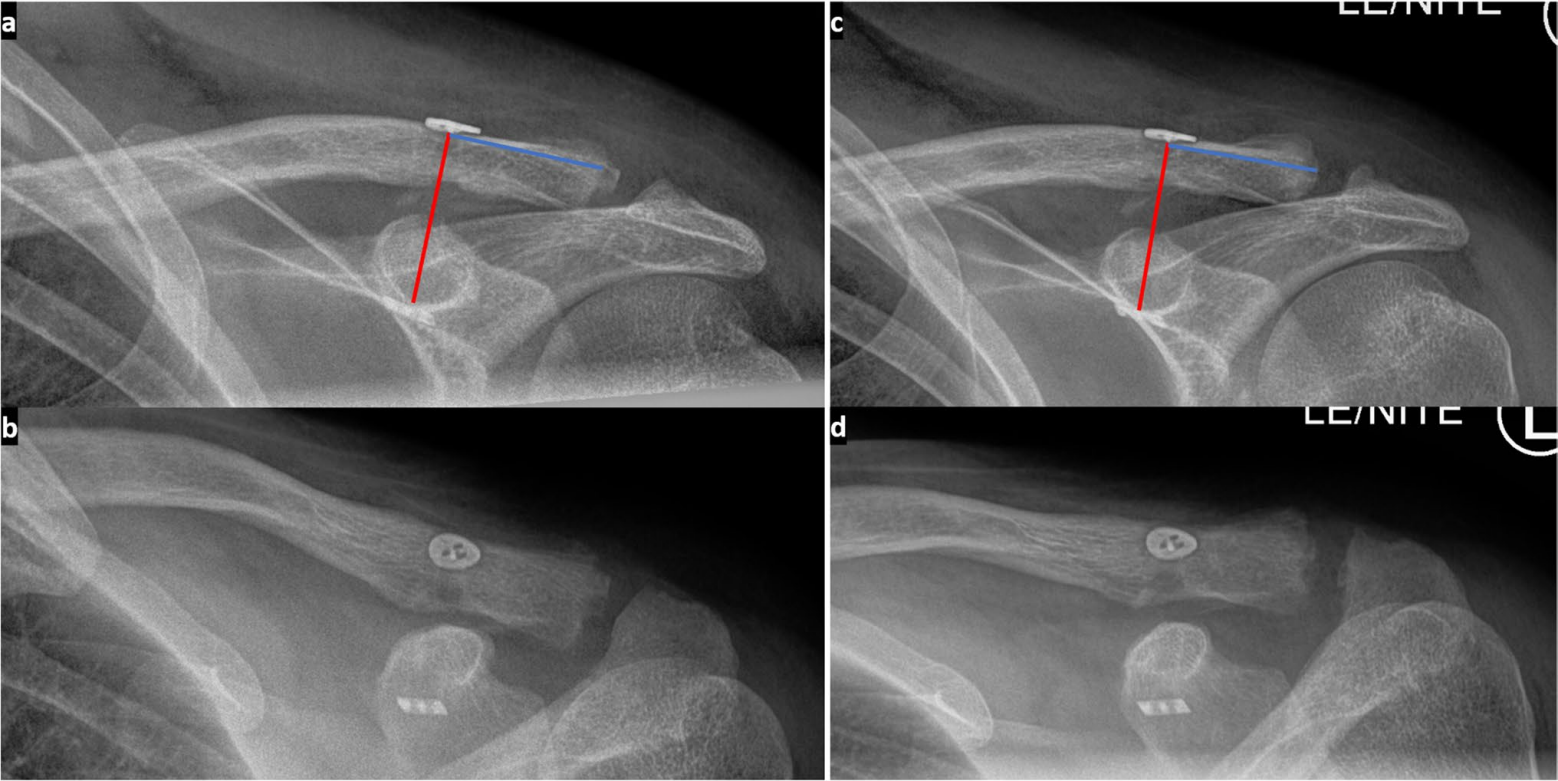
The collection of radiological measurement data is demonstrated here on two X-ray controls in two planes each. The anterior posterior (a.p. / coronal) AC joint images at discharge (a) and after 3 months (c) show the measured CC distance (vertical AC joint congruence) between the titanium plates in mm (red line in each case) and the measured distance from the center of the clavicle drill hole to the lateral end of the clavicle in mm (blue line in each case). In the second plane (b at discharge and d after 3 months in oblique / axial), an assessment of the horizontal AC joint congruence is possible
